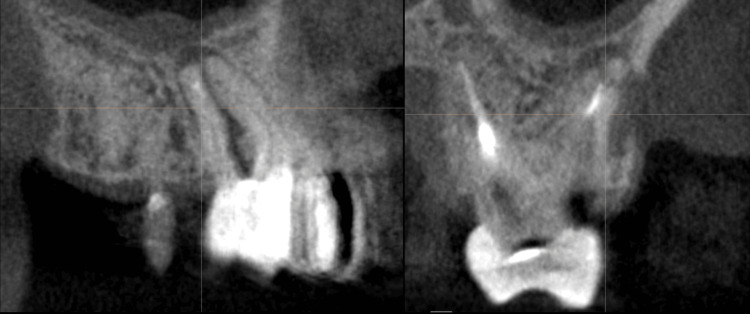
Cone-beam computed tomography (CBCT) showing extensive bone loss in the region of tooth 16 (upper right)
